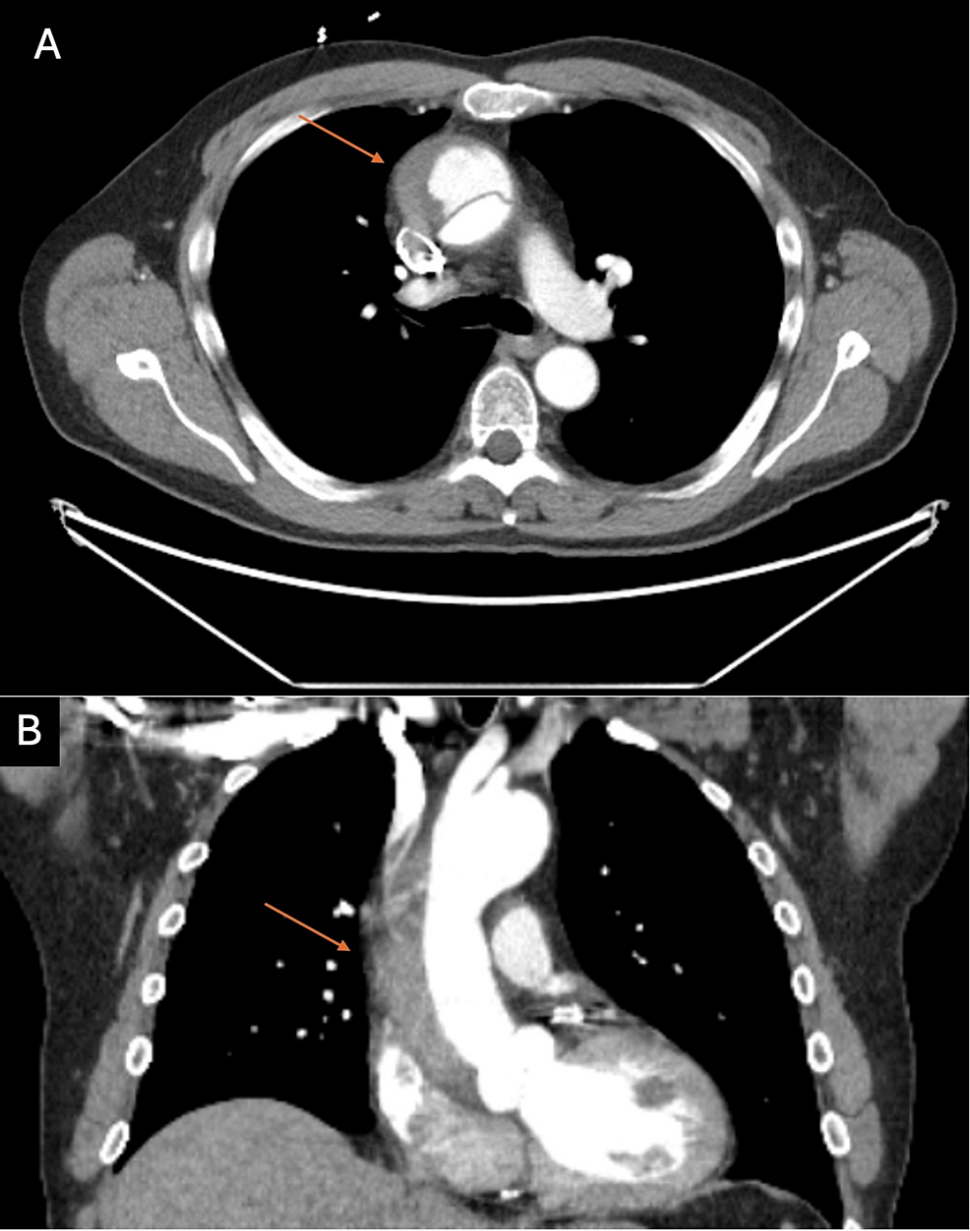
CT angiogram with type A aortic dissection with aneurysmal dilation of 5.3 x 5.4 cm at maximal diameter. False lumen on the patient's right (orange arrows) and true lumen opacified with contrast. Panel A: Transverse axis view of TAAD extending from the aortic root up to the distal aortic arch with intimal tears noted within the arch. Panel B: Coronal axis view of TAAD.
